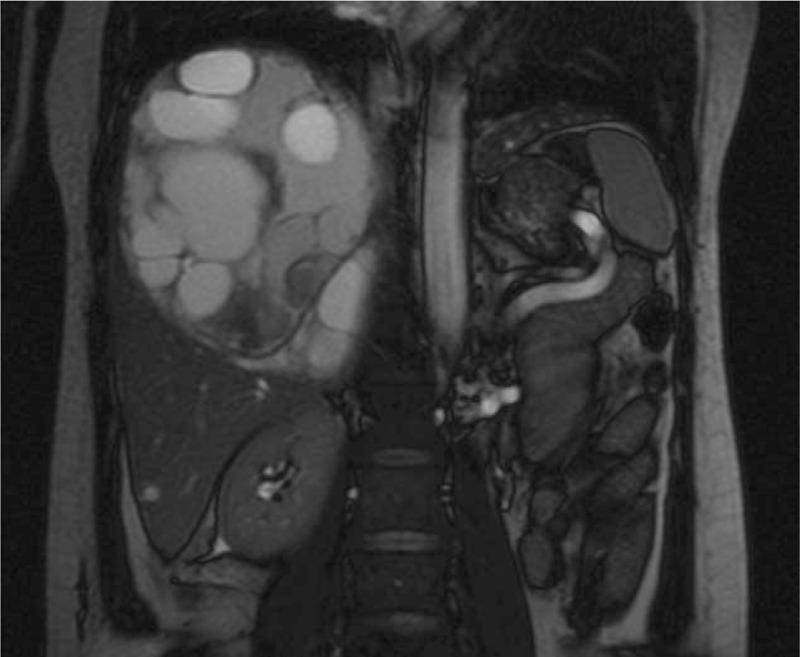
MRI showed a large cystic lesion containing smaller round shaped cystic lesions with hemorrhage and fluid. MRI = magnetic resonance imaging.
